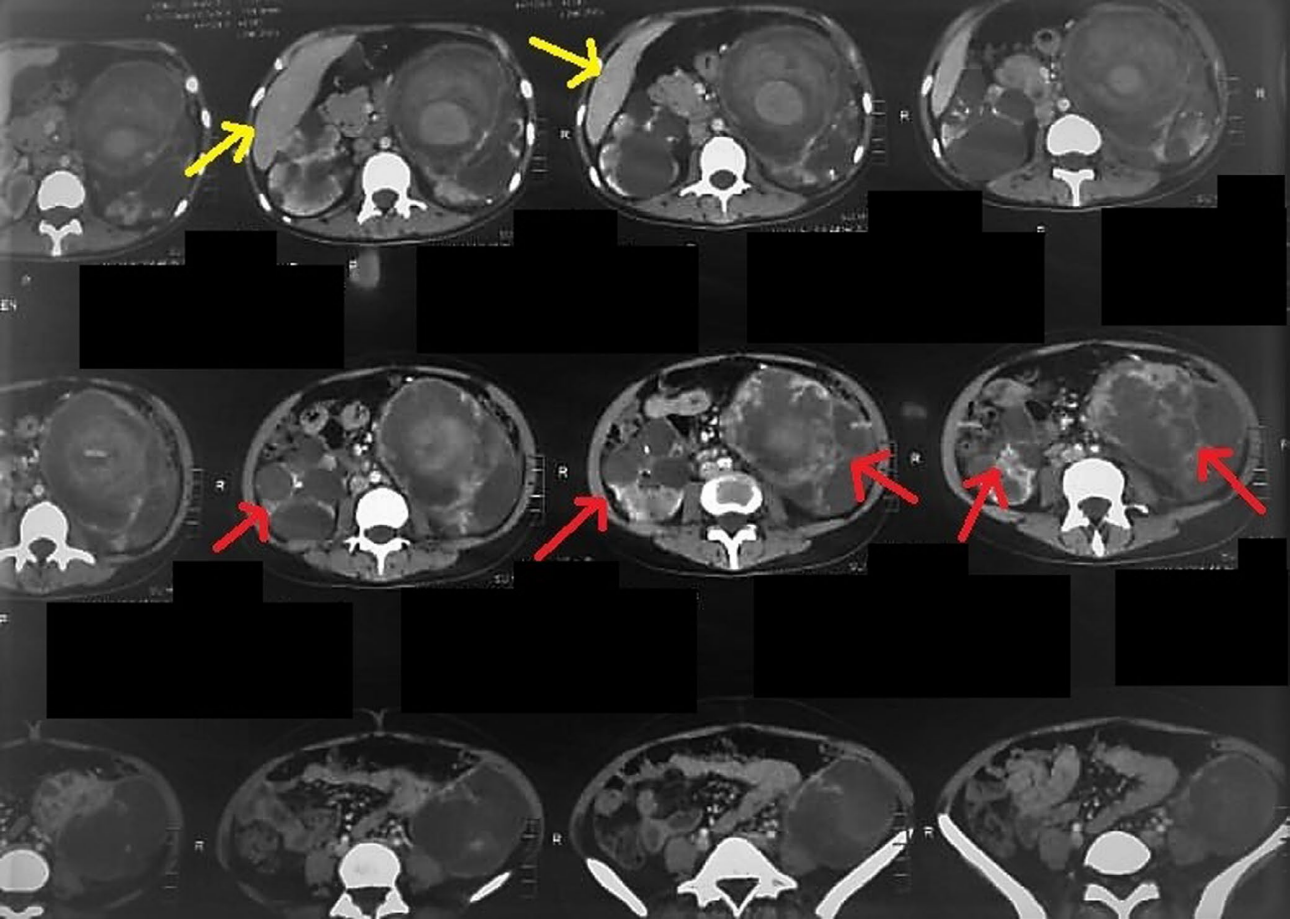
CT abdomen and pelvis shows bilateral renal cystic lesions, suggesting polycystic kidney disease (red). Additionally, large pseudoaneurysm formation on left side can be seen (yellow)
